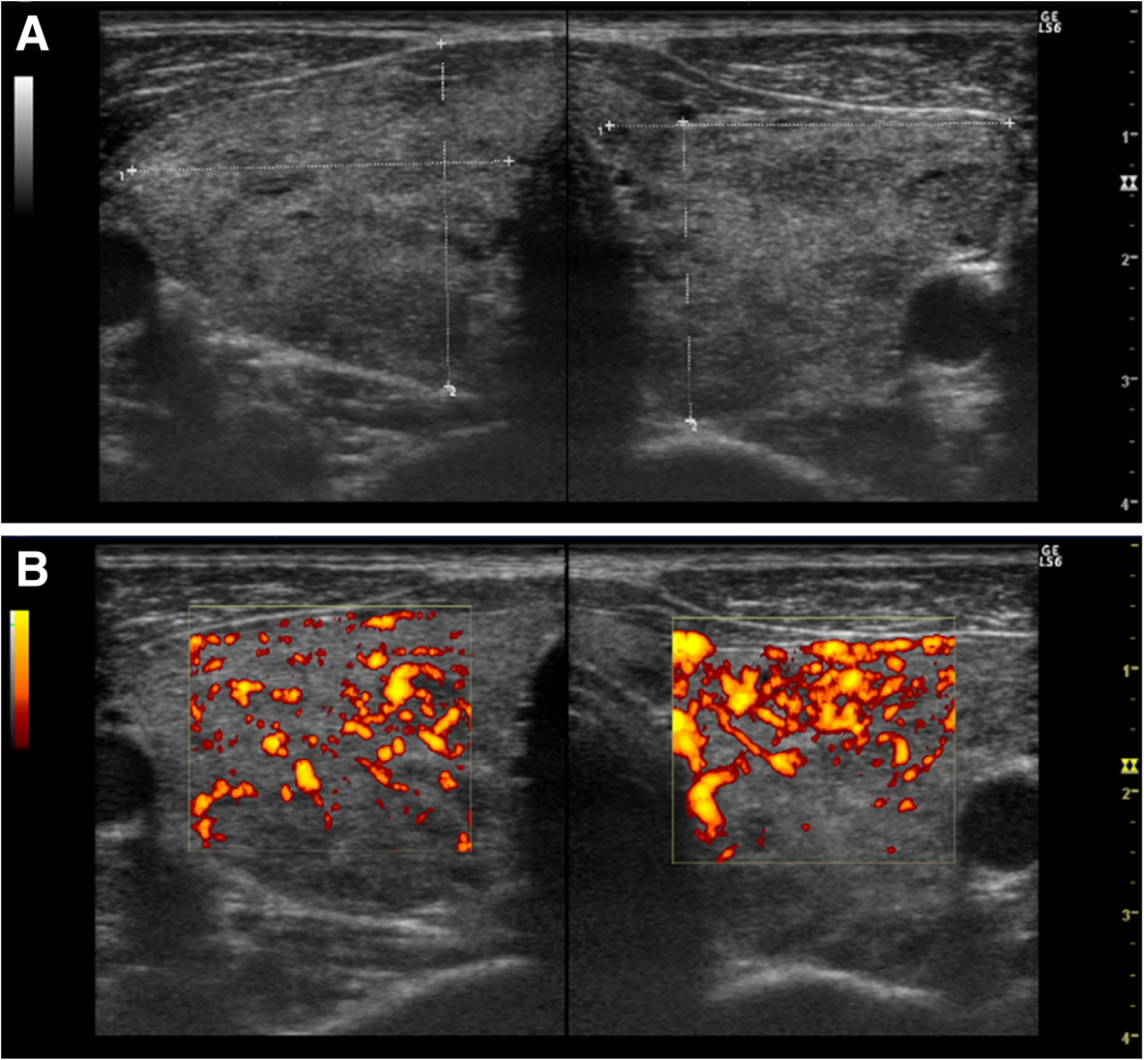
Ultrasonography of the thyroid gland (July 2013). a Ultrasonography showing heterogeneous and low echogenicity in a diffusely enlarged thyroid gland. No tumor was detected in the thyroid gland. b Color flow Doppler imaging revealing increased blood flow
